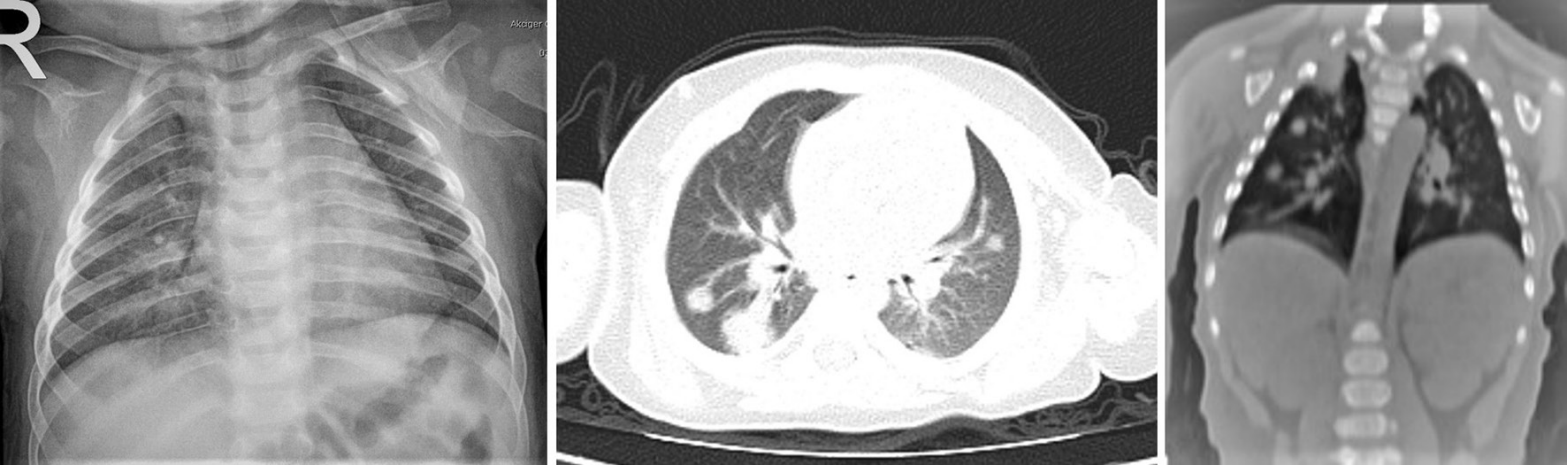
Male, 6- month-old. Chest X-ray and CT showed multiple round-shaped GGO with round multiple consolidation areas.
